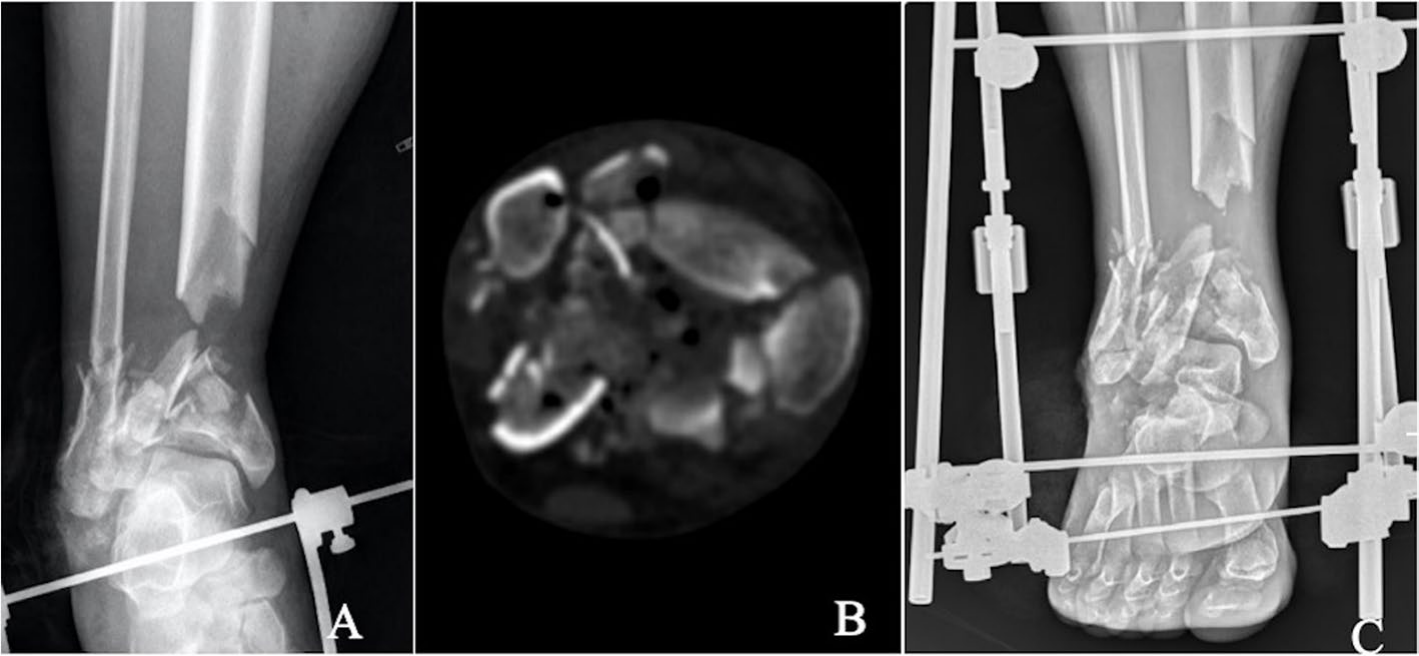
Anteroposterior (A), and computed tomography (CT) scan (B) of a middle-aged patient who fell from a height and sustained a type IIIA open AO/OTA type C3.3 pilon fracture among other injuries. Anteroposterior (C),immediate debridement, spanning external fixation. The distal tibial metaphyseal defect was evident
